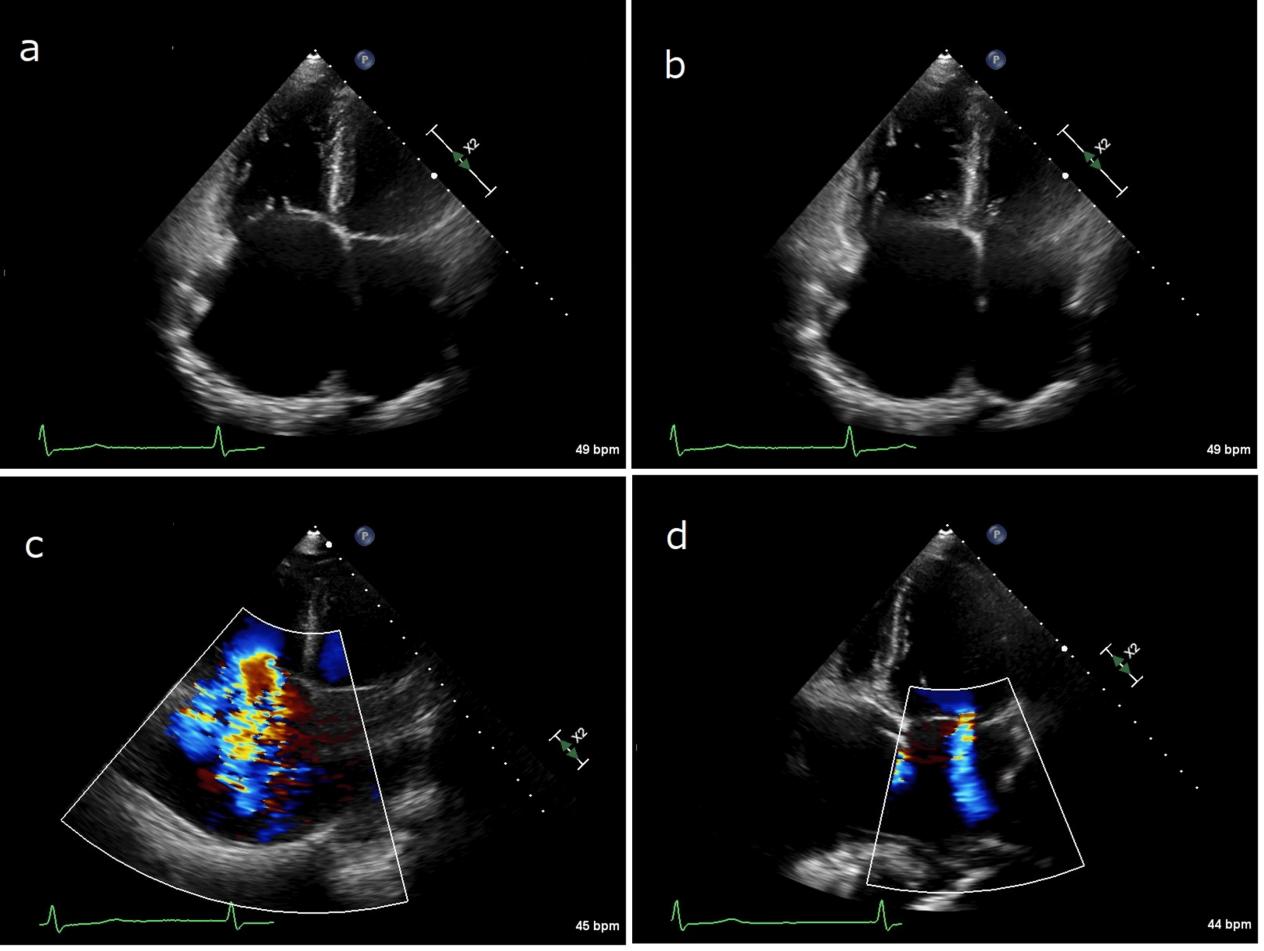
TTE on admission a: Systolic function; b: Diastolic function. Both TTEs (a and b) showed dilation of both atria, particularly the RA, and a markedly dilated tricuspid annulus with loss of coaptation of the valve leaflets; atrial contraction was absent. c: A Doppler study on admission showed severe TR; d: A Doppler study on admission showed trivial MR TTE: Transthoracic echocardiography, RA: Right atrium, TR: Tricuspid regurgitation, MR: Mitral regurgitation
